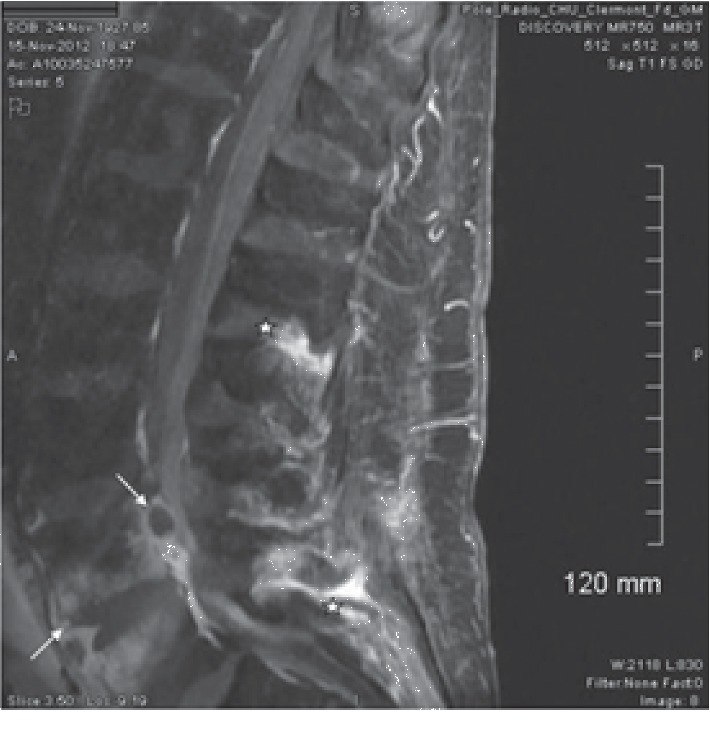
MRI of the lumbar spine (T1 fat sat + gadolinium): the gadolinium-enhanced sagittal T1-weighed MR image shows peripheral enhancement of L4-L5 disc and marrow edema extending from the L4 and L5 vertebrae with anterior epidural abscess-like spondylodiscitits (arrow). The MR image shows inflammation with enhancement of L1-L2 and L4-L5 zygapophysial joints (stars).
